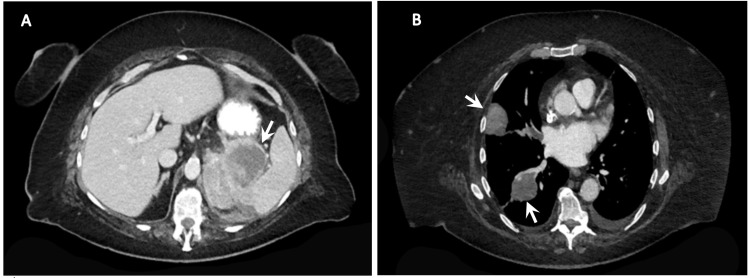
(A) Mass in the left nephrectomy bed with invasion into the splenic vasculature. (B) Presence of metastatic disease to the lungs. CT of the chest, abdomen, and pelvis with contrast demonstrating metastatic spread of renal cell carcinoma into the left nephrectomy bed and splenic vasculature and spread into the lungs.
